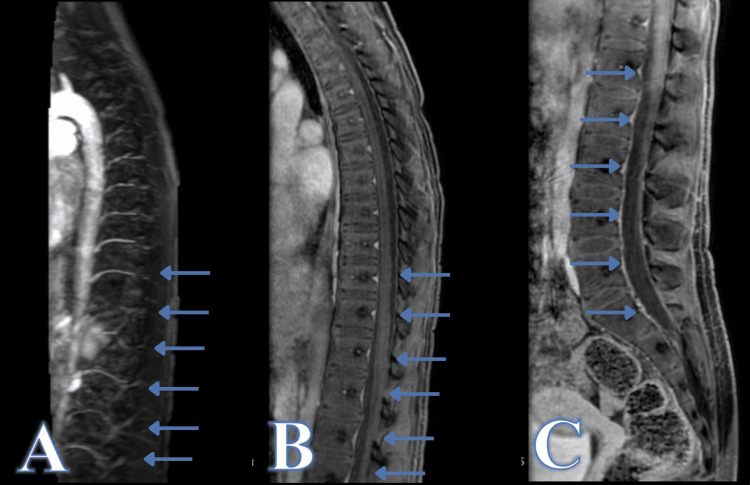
Magnetic resonance imaging demonstrating hematoma resolution and absence of vascular malformations Sagittal image of spinal magnetic resonance angiography (MRA) with IV contrast (A), sagittal T1 with fat suppression and IV contrast at the level of the thoracic spine (B) and sagittal T1 with fat suppression and IV contrast at the level of the lumbar spine (C).
